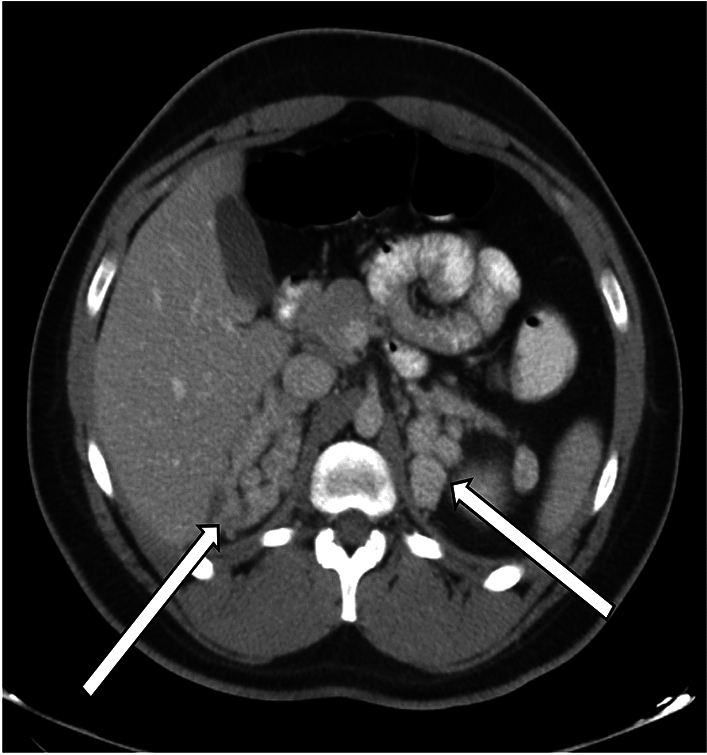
A representative case of CAH in a 21-year-old man, with axial CT showing nodular thickening of both adrenal glands (arrows)
